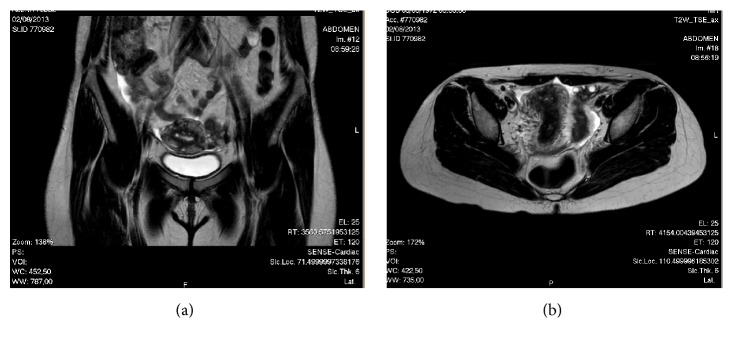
MRI: T2-weighted imaging. (a) Coronal view. (b) Axial view: uterus with multiple small nodules regarded as leiomyomas and with some endometrial glands suggesting adenomyosis.
